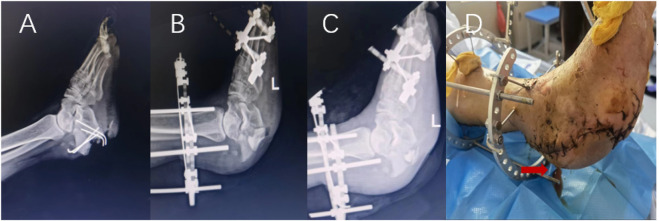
Male patient, 59 years old, X-ray before operation (A), X-ray 1 day after operation (B), X-ray 1 month after operation (C), serous exudation appearance of incision on the 5th day after operation (D).
